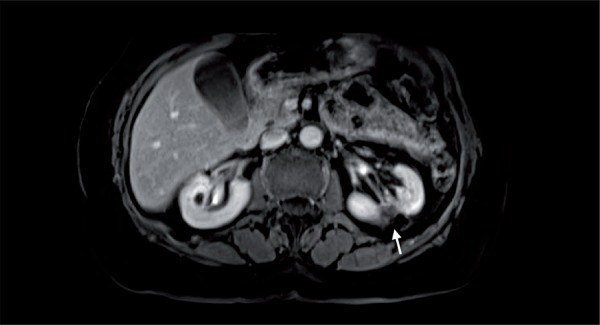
MR image, T1 post-contrast for surveillance two years post ablation. Arrow: Retracted and non-enhancing ablation zone, depicting successful treatment.
